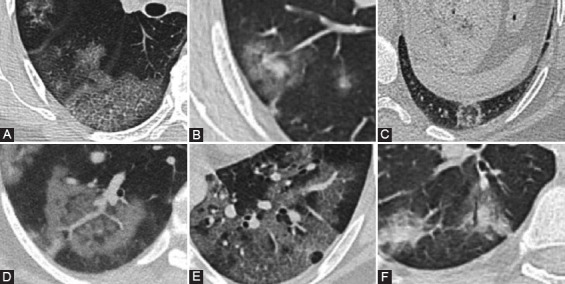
Computed tomography features of coronavirus disease 2019 pneumonia. (A) Reticular pattern. (B) Halo sign (Ground-glass opacities with centrally consolidation). (C) Reverse halo sign (a ground-glass opacity area in the center and completely surrounded by high-density consolidation). (D) Vascular enlargement. (E) Air-bubble sign. (F) Air bronchogram appear on the background of consolidation and ground-glass opacities.
